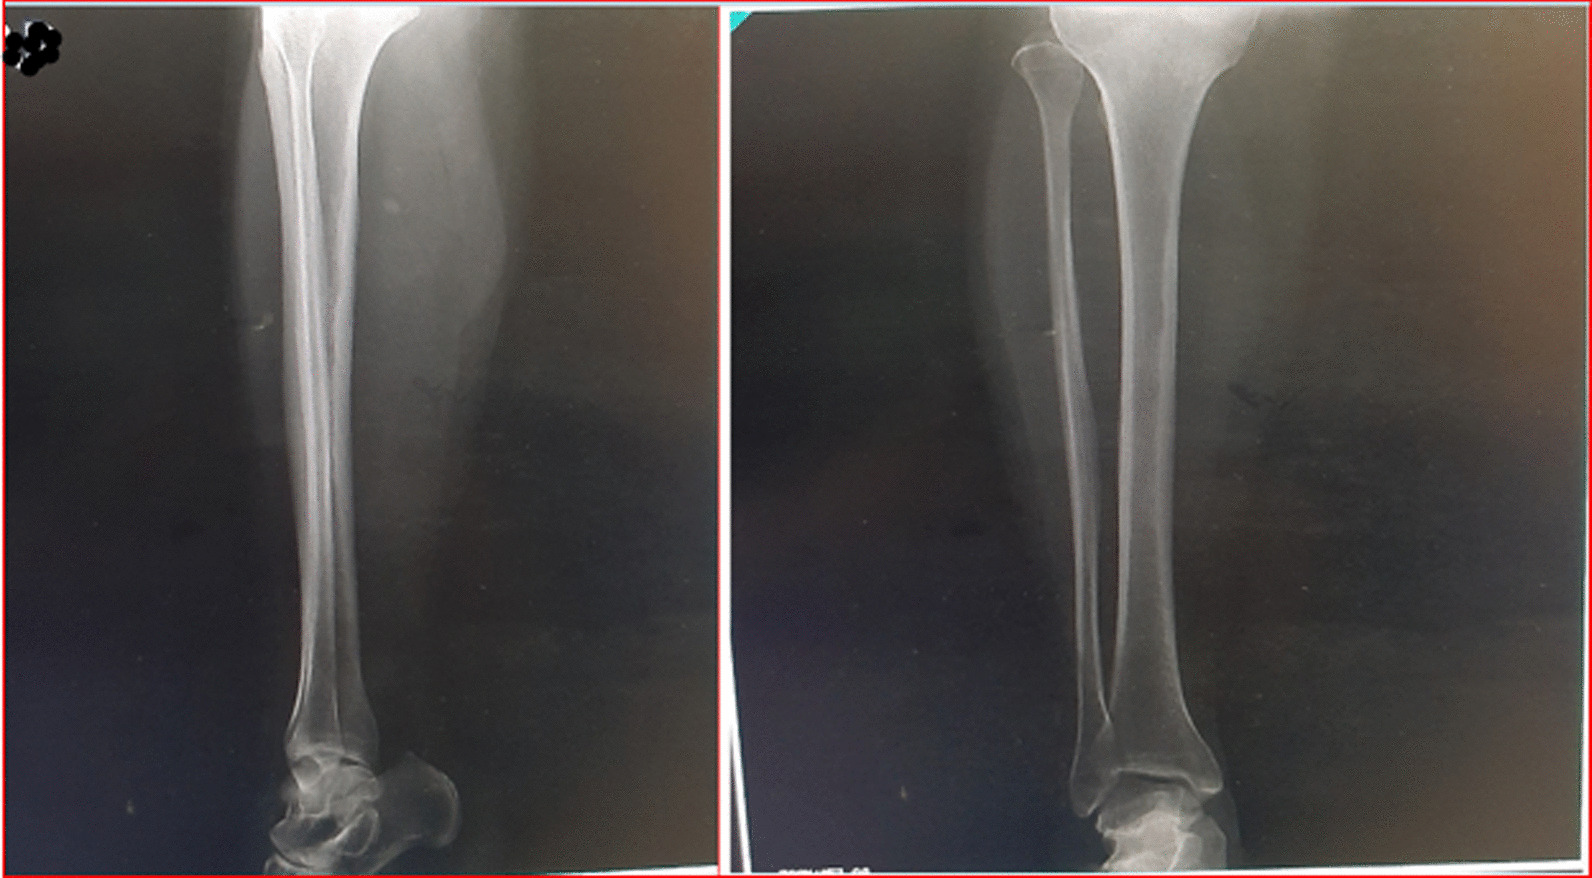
Anterior/posterior and lateral radiographs of the right tibia bone
